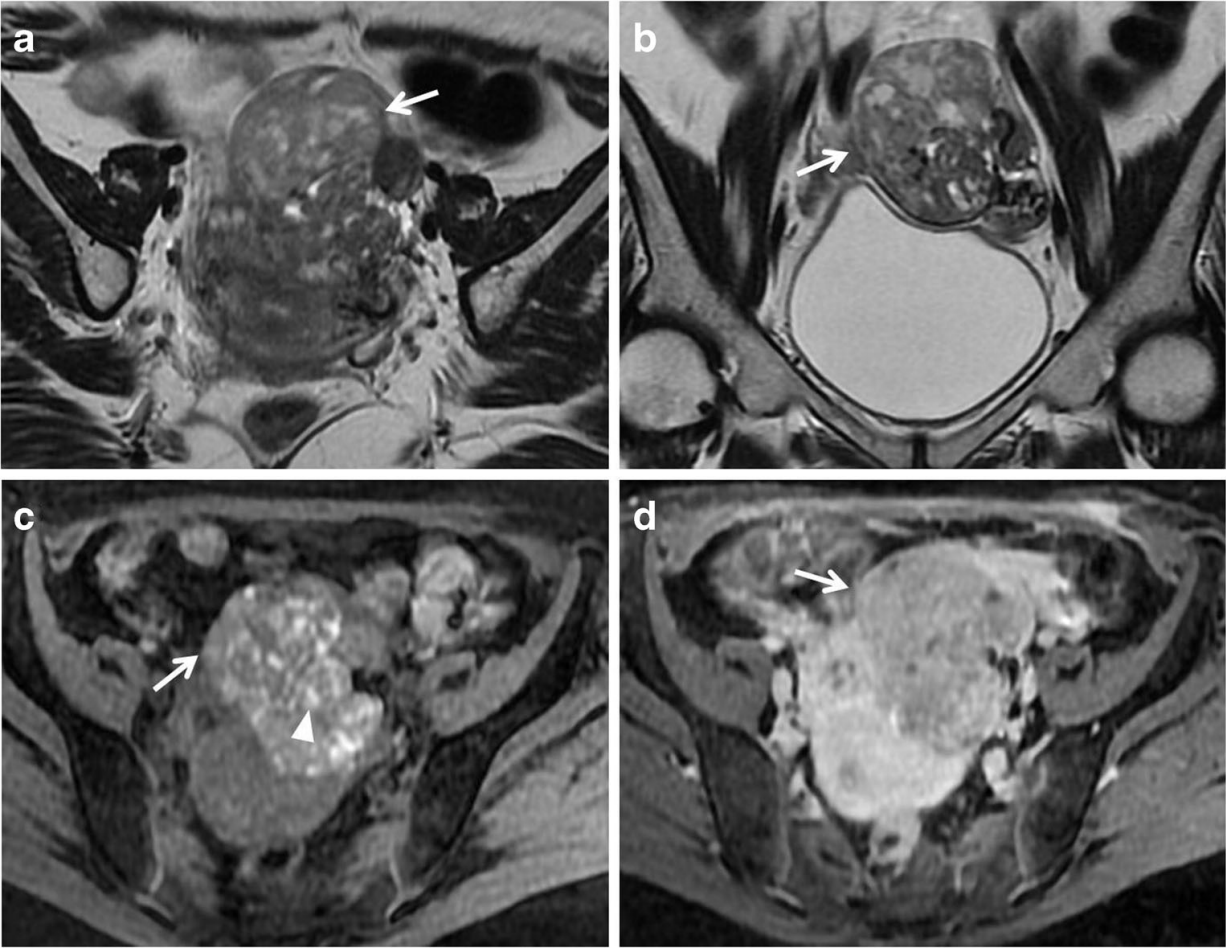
Struma ovarii in a 32-year-old woman. (a) Axial T2-weighted, (b) coronal T2-weighted and (c) axial fat-suppressed T1-weighted images show a predominantly solid mass (*white arrows*) with multiple cystic spaces of variable signal intensity, some of which show high signal intensity on T1-weighted image (*white arrowhead*). (d) Axial contrast-enhanced fat-suppressed T1-weighted image demonstrates enhancement of the mass (*white arrow*)
